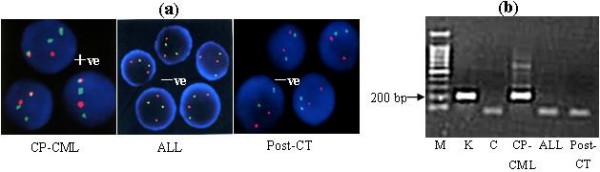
The results of FISH and RT-PCR analyses of marrow samples aspirated during different disease stage in a patient with CML. CP-CML, the sample from chronic phase (October 2000); ALL, from the disease stage of acute lymphoblastic leukemia (October 2006); Post-CT, from post-induction chemotherapy for ALL (December 2006). + ve, BCR/ABL positive; - ve, BCR/ABL negative. (a) FISH analysis; (b) RT-PCR analysis. Lane M, 100 bp molecular weight ladder; Lane K, K562 200 bp b3/a2 BCR/ABL positive control; Lane C: negative control; Lane CP-CML, 200 bp b3/a2 products; Lane ALL and Post-CT, BCR/ABL negative.
